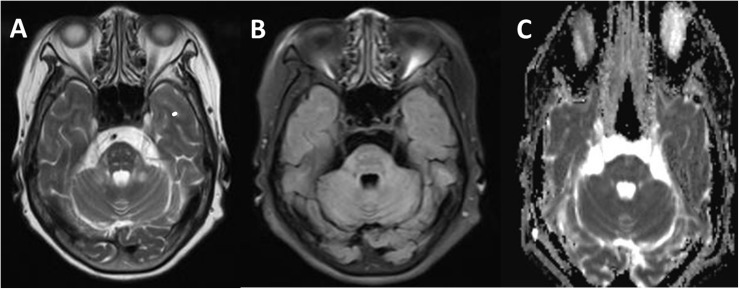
Comparison of a T2W (A), fluid-attenuated inversion-recovery (FLAIR) (B), and apparent diffusion coefficient (ADC) (C) in transverse section of the pituitary gland. Signal alterations were extended to pyramidal tracts, corticonuclear tracts, medial lemniscuses, and the degustation of the superior cerebellar peduncles (A). These signals above lesion areas were suppressed on FLAIR and not affected on the ADC map (C).
